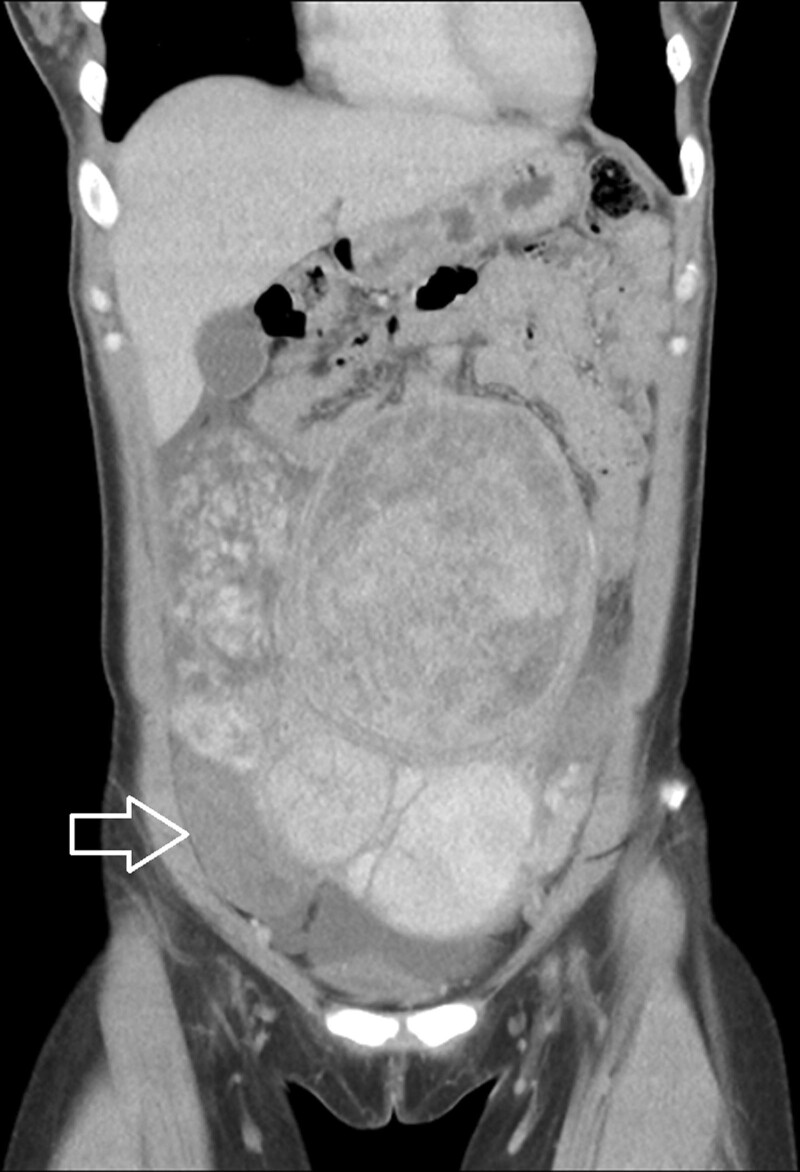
Coronal view of CT imaging with contrast enhancement showed a poor enhancement subserosal leiomyoma (arrow). CT = computed tomography.
